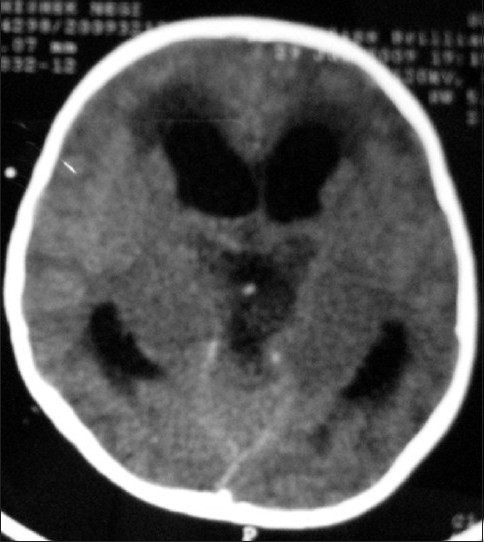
Axial section of noncontrast CT scan of head on post operative day 5 after the removal of external ventricular drain, showing enlarged size of ventricle
